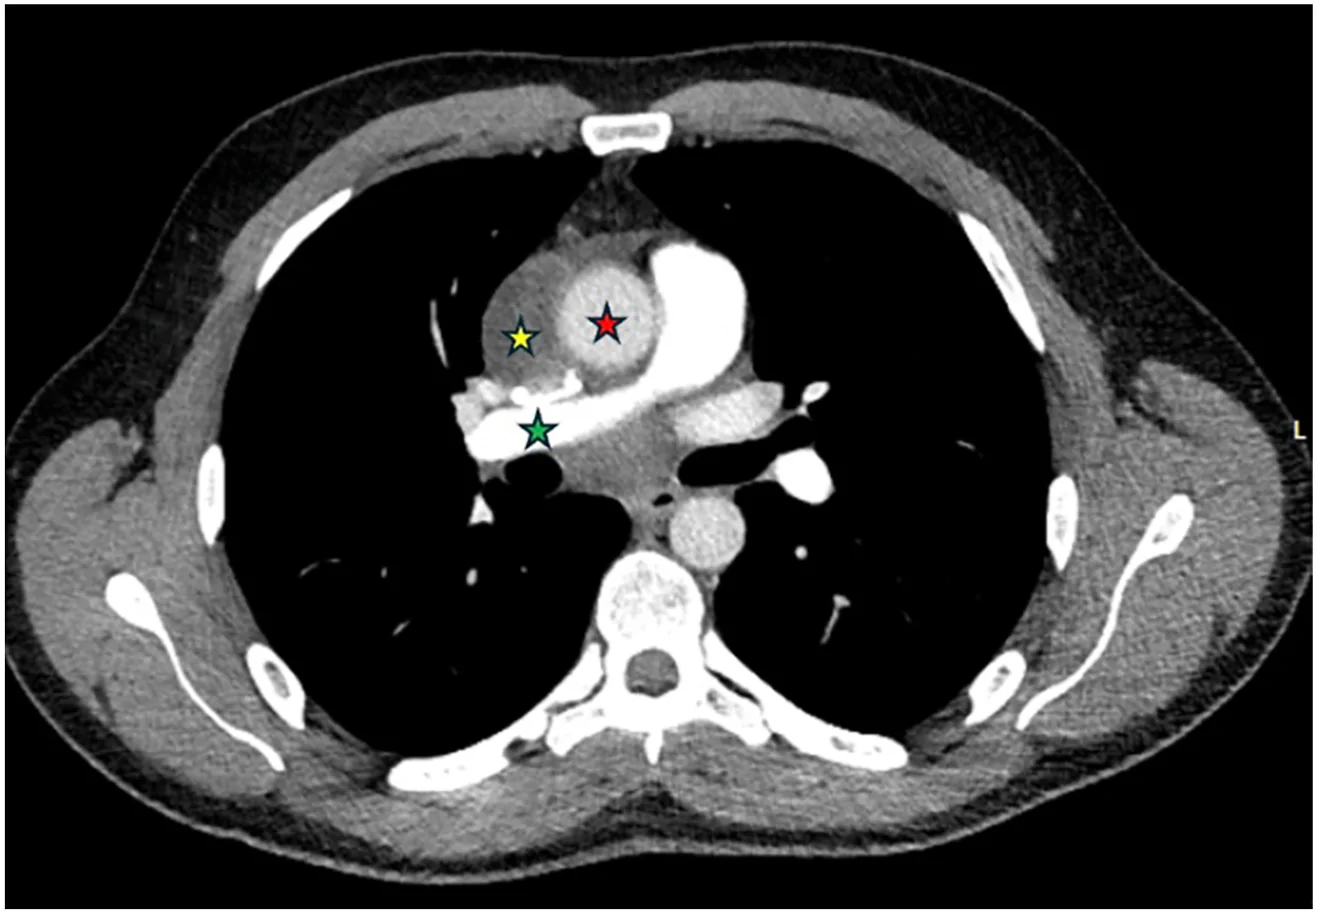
Chest computed tomography with a yellow star identifying the lesion abutting the lateral aspect of the ascending aorta (red star) and anterior to the right pulmonary artery (green star).
